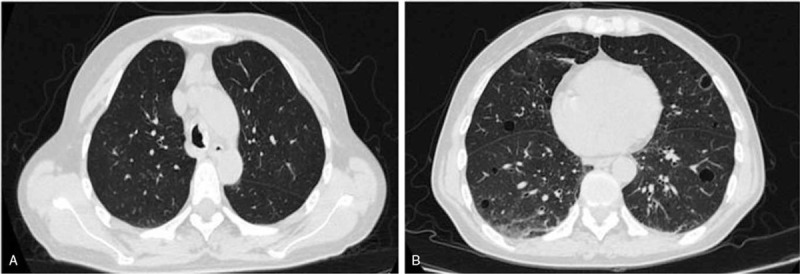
A. Chest CT scan revealed the mass in the lumen of trachea. B. Ground glass shadows, thin walled cavity and multiple nodule shadows.
